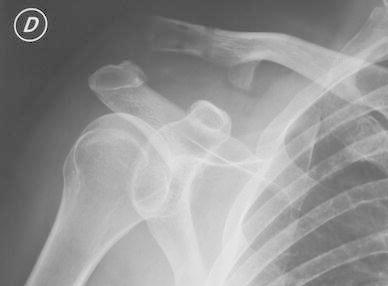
Postoperative X-rays: complete dislocation after stabilization with the biological graft. Note the coracoclavicular ossification
